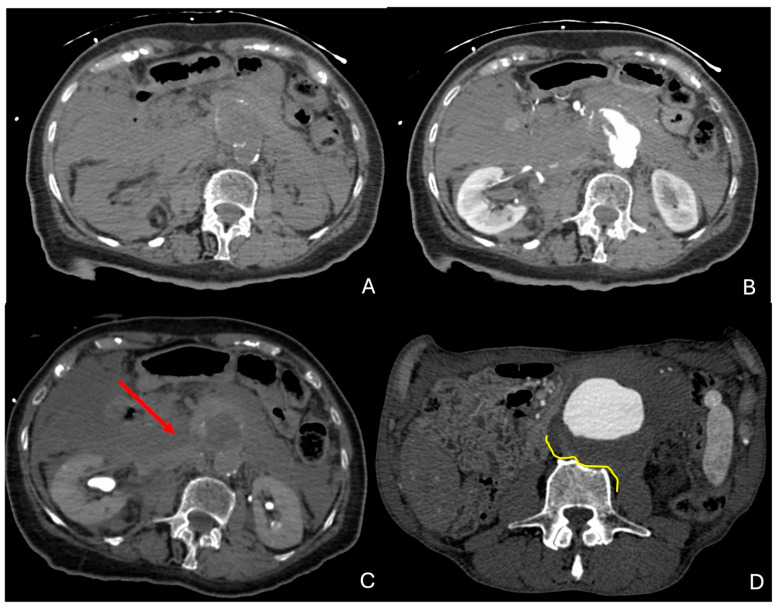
Signs of rupture of AAA. (A) Unenhanced CTA showing a large intra- and retroperitoneal hematoma with blood density in the context of a ruptured AAA of a patient admitted to the hospital for abdominal and back pain. (B,C) Arterial and delayed phase CTA showing contrast extravasation of the ruptured AAA in the same patient (red arrow). (D) Draped aorta (yellow line) with slight scalloping of the adjacent vertebra, in the context of a contained rupture in the retroperitoneum of another patient. CTA: computed tomography angiography; AAA: abdominal aortic aneurysm.
